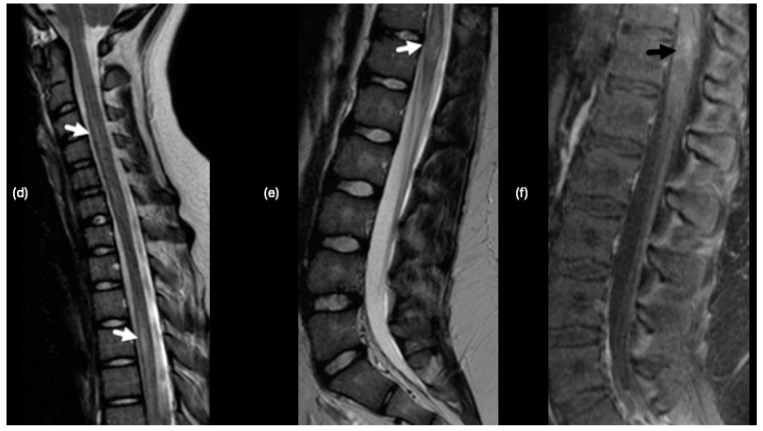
A 10-year-old female presenting with right monocular vision loss. (a) Coronal T2 orbital image demonstrates abnormal edema and swelling of the intraorbital right optic nerve (white arrow), as well as abnormal enhancement (black arrows) of the (b) prechiasmatic (b,c) intraorbital right optic nerve. Six weeks later, the patient presents with new voiding and extremity weakness. On (d,e) sagittal T2-weighted images, there are ill-defined cord signal abnormalities (white arrows) with associated subtle enhancement (black arrow) of the distal cord on (f) sagittal T1-weighted post contrast fat saturated spine MR image.
